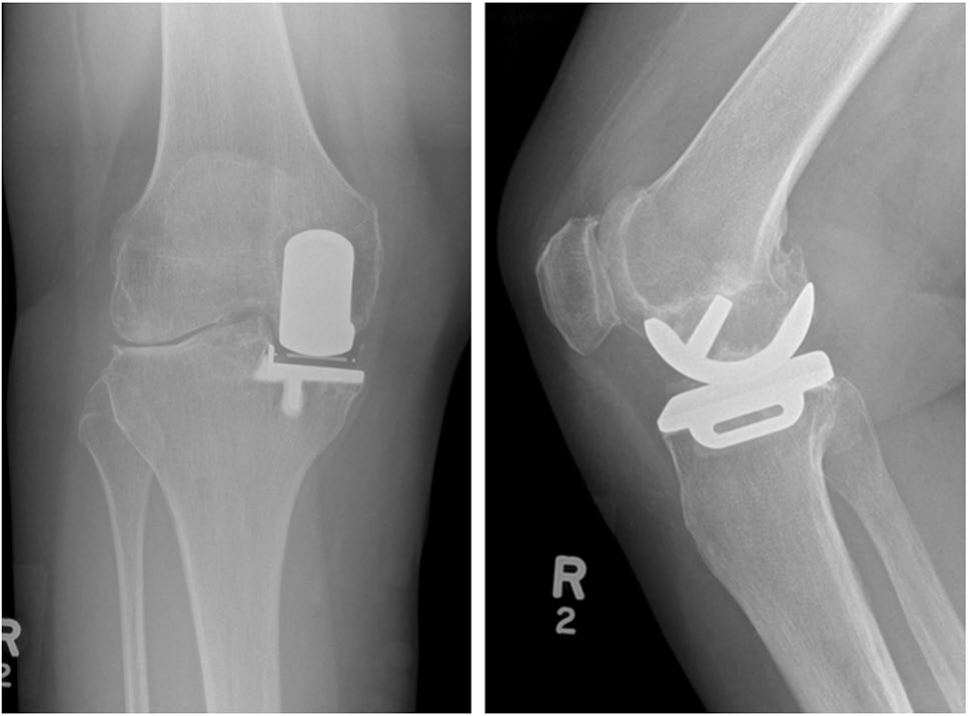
AP-X-ray (Left) and Sagittal-X-ray (Right) of medial OUKR with lateral osteoarthritis progression prior to conversion to bi-UKR
